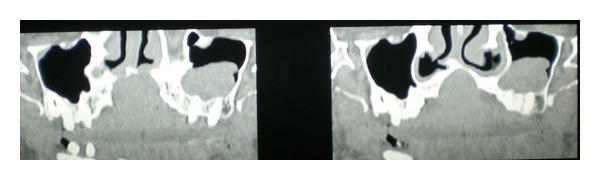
Panoramic reconstruction of CT scan.
